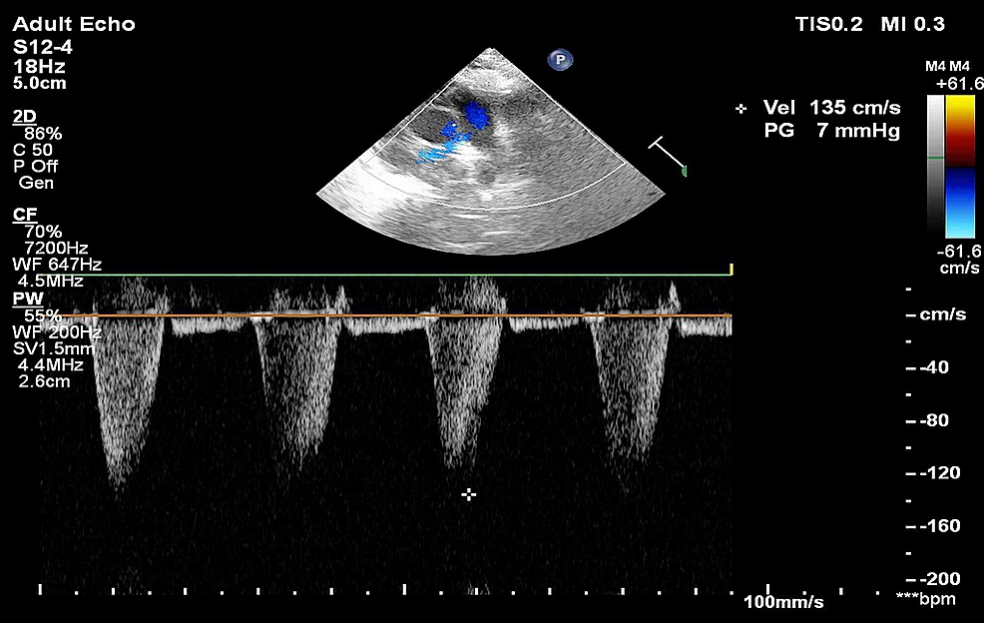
Color Doppler ultrasound of the right radial artery distal to the deployed stent shows a normal phasic pattern suggestive of a patent lumen with adequate flow.
